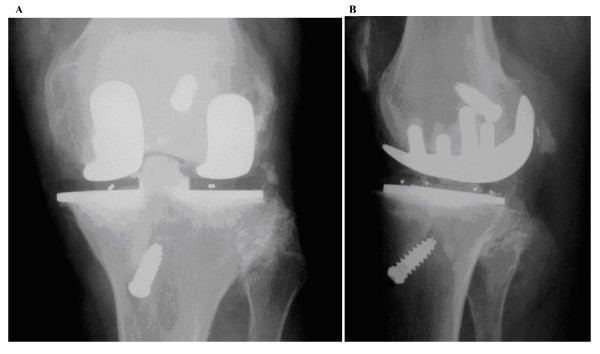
Radiograph of bi unicompartmental replacement with ACL reconstruction. A - Anteroposterior View, B - Lateral View.
